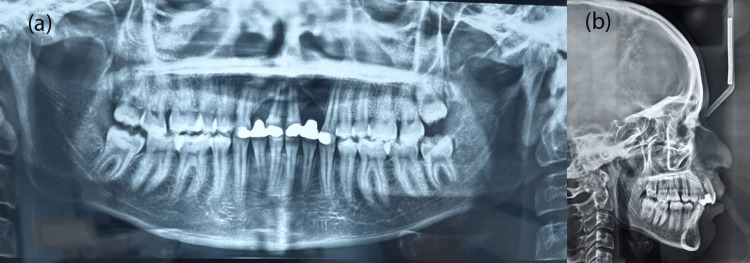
Post-treatment radiographs: (a) OPG, (b) lateral cephalogram.
